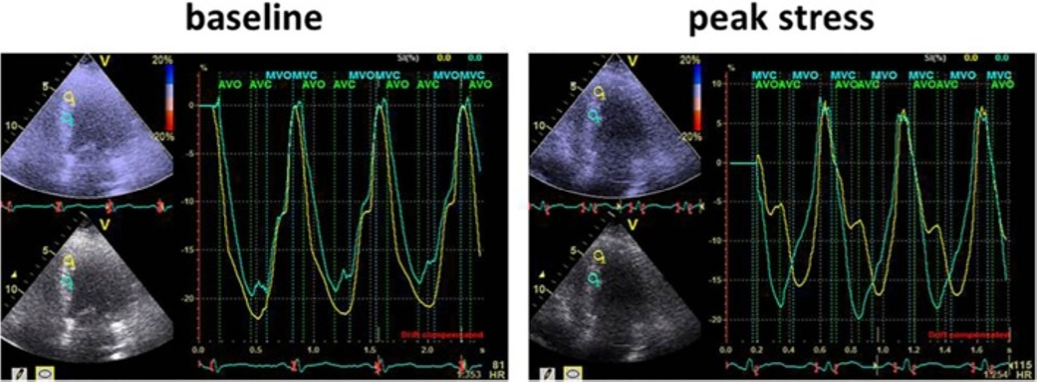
If there is any doubt about regional function, tissue Doppler strain is the fastest means of regional function analysis in a stress echocardiogram.
